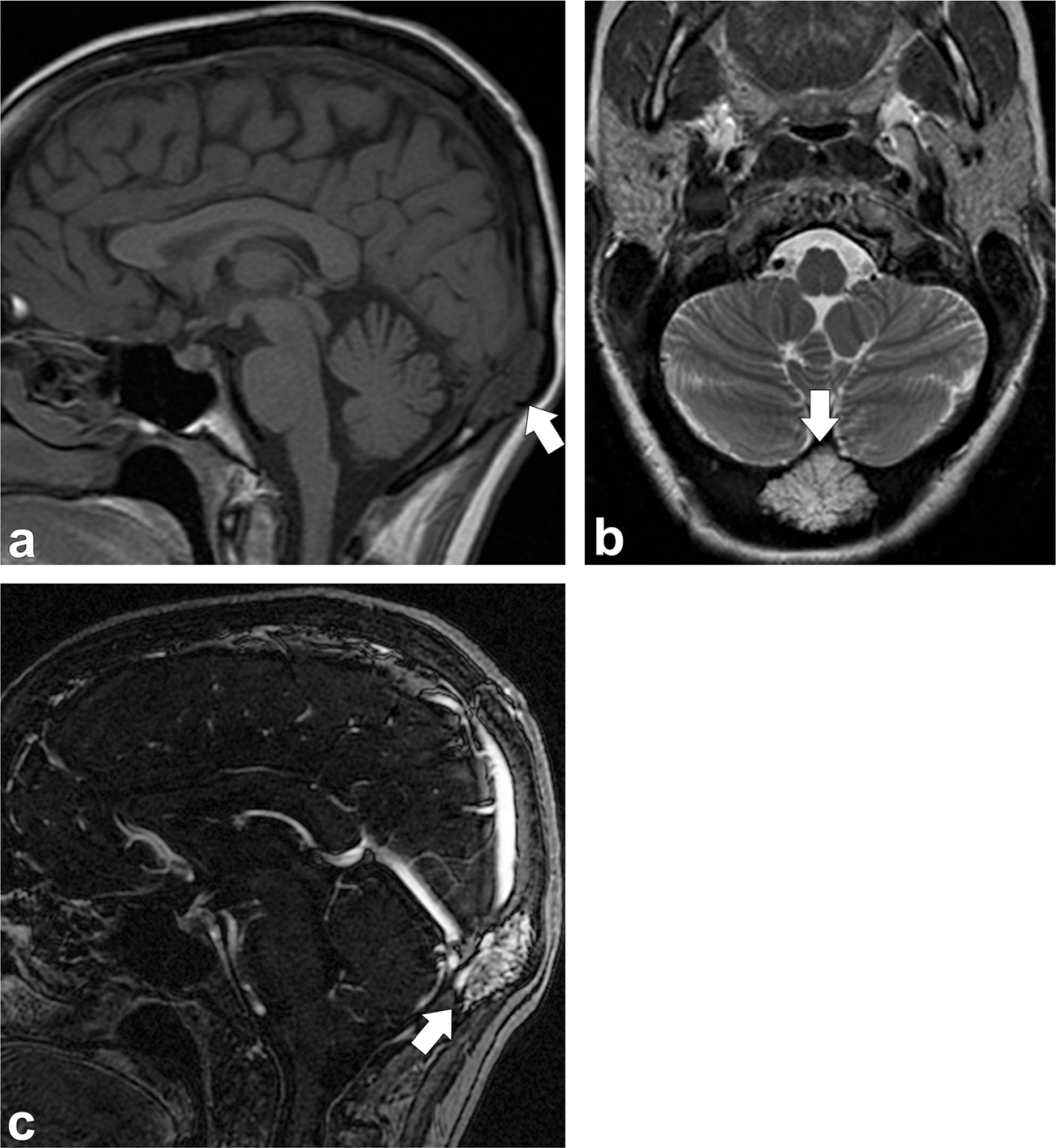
Osseous venous vascular malformation. Sagittal T1-weighted imaging (a), axial T2-weighted imaging (b) and sagittal contrast-enhanced venogram (c) show a hypointense T1, hyperintense T2 and enhancing lesion in the occipital bone (*arrows*)
